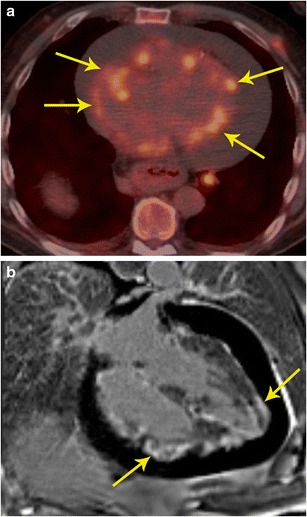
Local extension. a Axial FDG-PET/CT image in a patient with melanoma shows multiple hypermetabolic nodules on the cardiac surface (arrow). There is also pericardial effusion. b MRI was performed to better characterize the extent of these lesion, particularly to see whether there was myocardial involvement. Four-chamber delayed enhancement MRI shows the multiple pericardial masses extending to the myocardium. Diffuse heterogeneous myocardial enhancement (arrows) is seen, indicating diffuse infiltration
